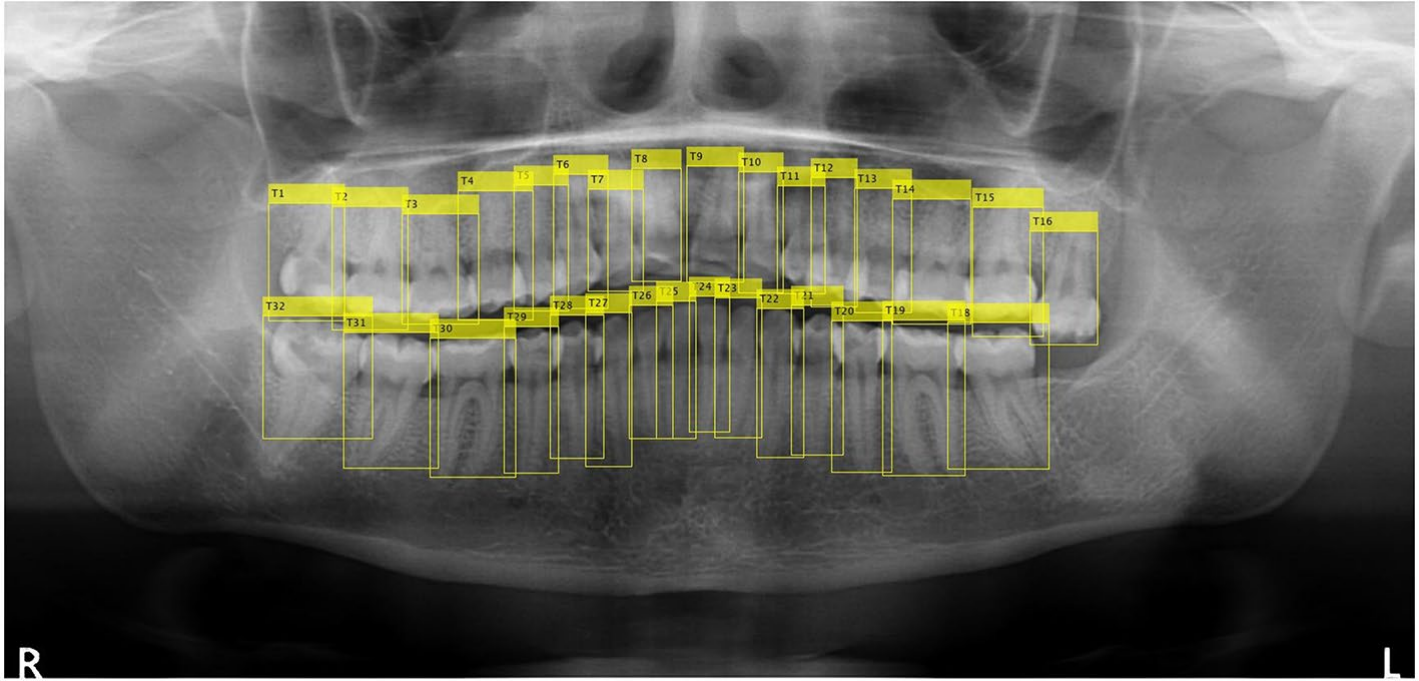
Successful teeth detection in noisy panoramic radiograph. The detected boxes were generated by MATLAB 2019a software.
